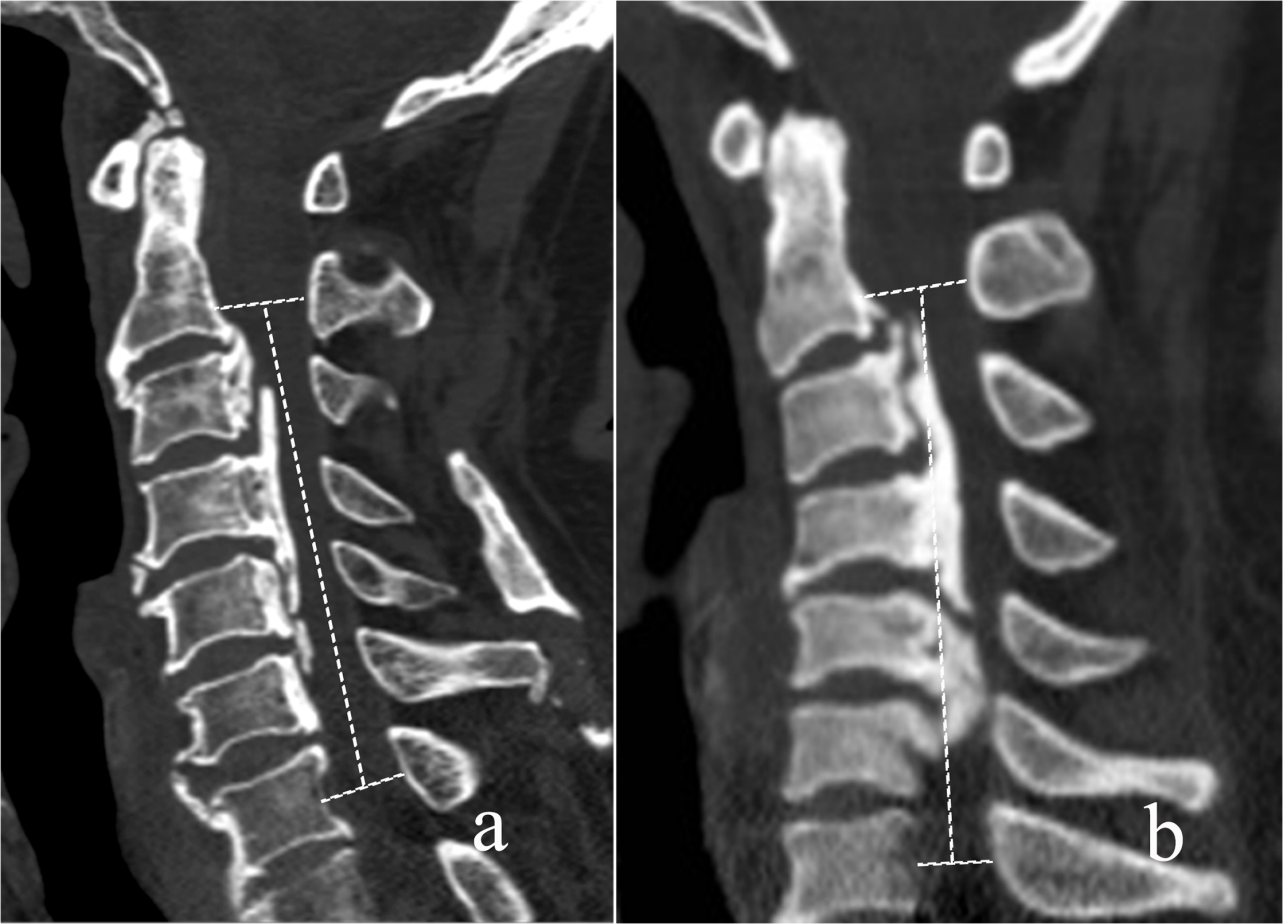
The K-line is a straight line that connects the midpoints of the spinal canal at C2 and C7 on the lateral cervical radiographs. Patients without OPLL exceeding the line were considered as K-line (+) ones (a) and those does exceed it were considered as K-line (-) ones (b).
